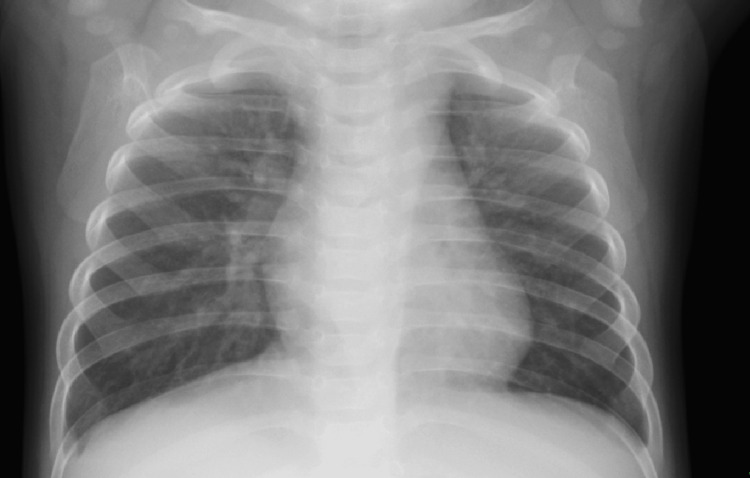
Chest radiography Chest radiography showed no cardiomegaly.
